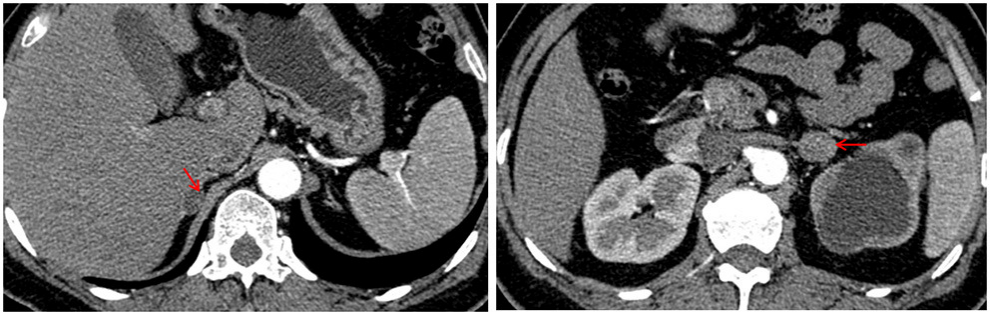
Adrenal enhanced computed tomography (CT) image showing a 16 × 13 mm right adrenal tumor and a 20 × 18 mm left adrenal tumor (red arrows).
